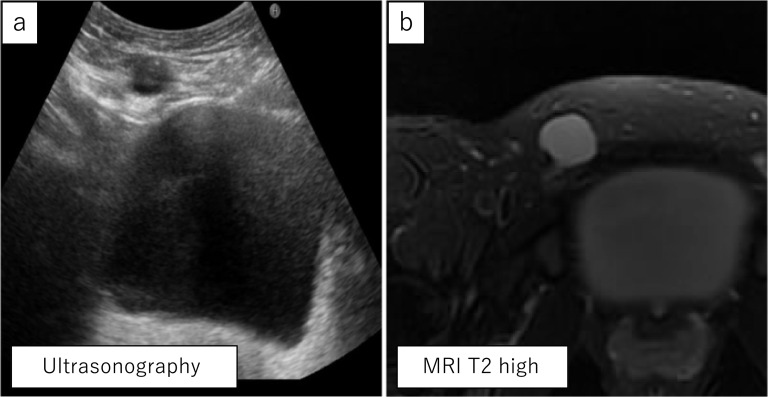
a Ultrasonography showed 3.0 × 2.0 cm cystic lesion. b Magnetic resonance imaging showed a 3.5 × 2.5 cm mass with high intensity on T2-weighted imaging in her right inguinal canal
